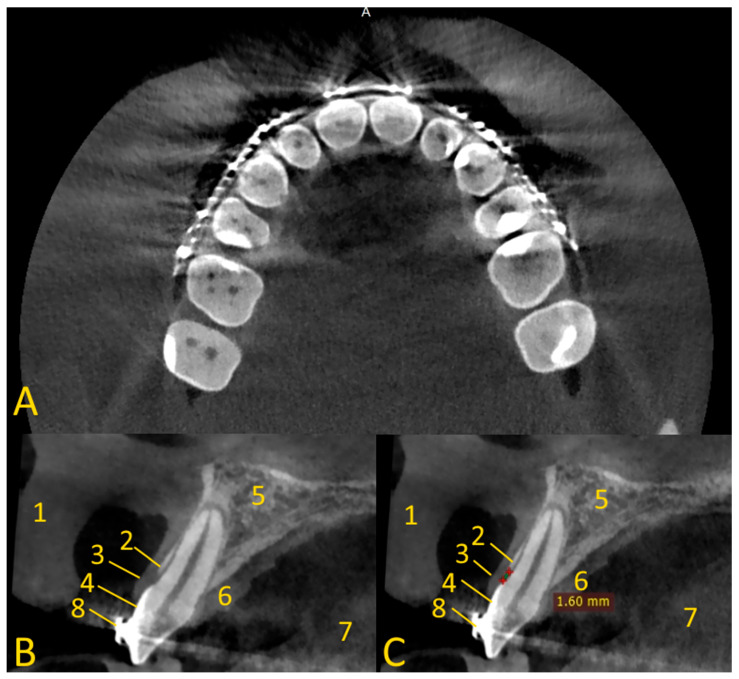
Soft tissue retraction maneuver in cone beam computed tomography in patient with orthodontic appliance (numerous artifacts are visible). Cases of soft tissue retraction, after viewing the CBCT images, can be assessed to clearly distinguish between the gingiva and the soft tissue on the palate. In cases where soft tissue retraction is not available, we are unable to clearly differentiate between the lip and gingiva: (A) axial view—lip retraction using cotton rolls; (B) cross-sectional view, tooth 12—enamel is under gingiva; (C) distance between the CEJ and the edge of the alveolar bone is very short. 1—lip; 2—bone edge margin; 3—gingiva; 4—enamel; 5—alveolar bone; 6—soft tissue of palate; 7—tongue; 8—metal orthodontic bracket.
